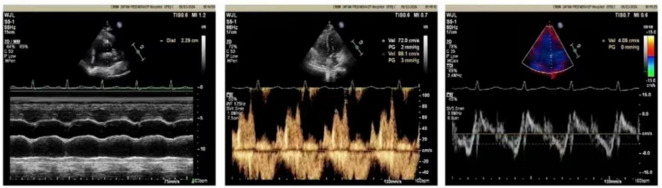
Follow-up echocardiographic images, demonstrating normal cardiac chamber size and preserved ventricular systolic function, with no significant valvular abnormalities.
